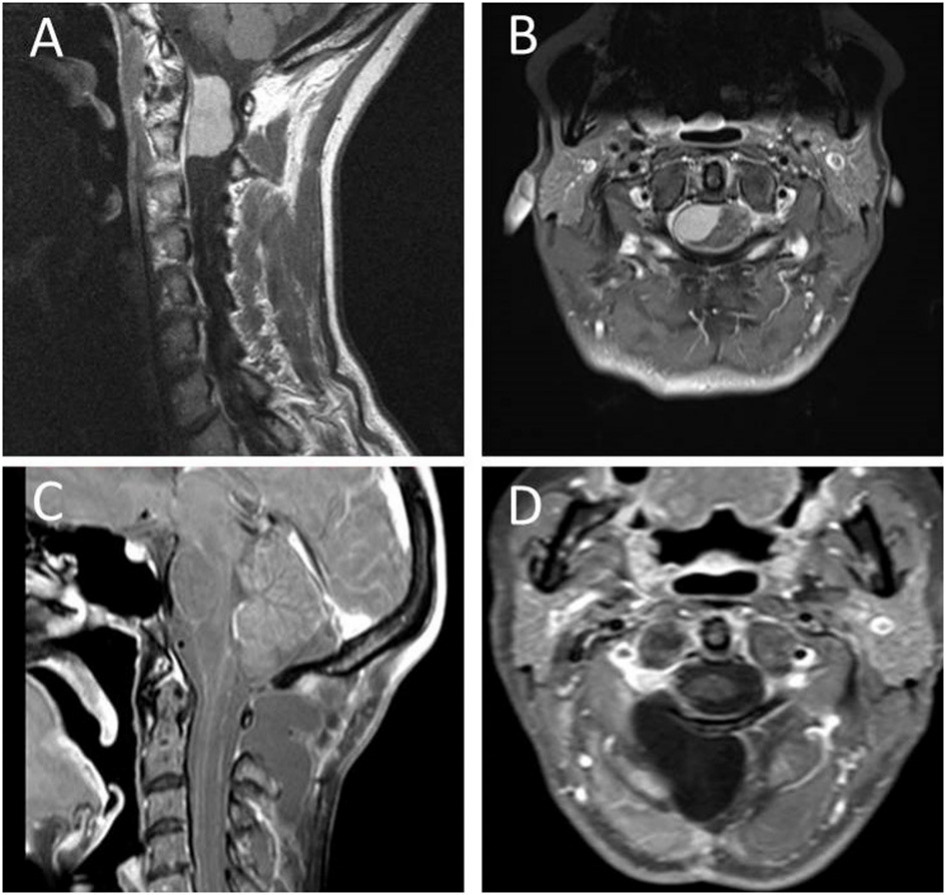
Preoperative sagittal (A) and transverse (B) T1-weighted contrast enhanced MRI of case 1 depicting an anteriorly located meningioma of the upper cervical spine with cranial extension into the foramen magnum. Postoperative sagittal (C) and transverse (D) T1-weighed contrast enhanced MRI showing complete resection of the WHO °II meningioma without the need of resecting any boney structures.
